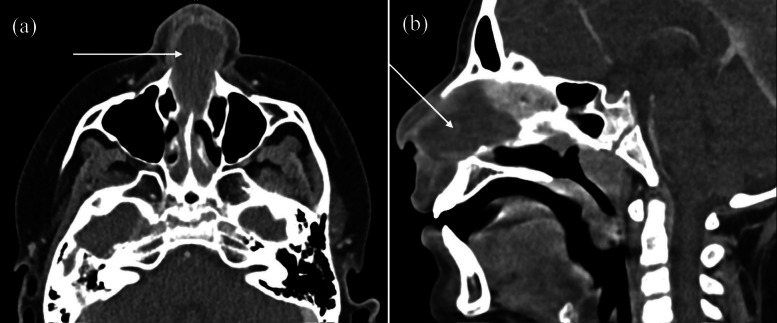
Axial (a) and sagittal (b) images of contrast-enhanced maxillofacial computed tomography demonstrating a large midline rim-enhancing, fluid-filled mass at the anterior nasal septum (arrows) with obstruction of both nasal cavities.
